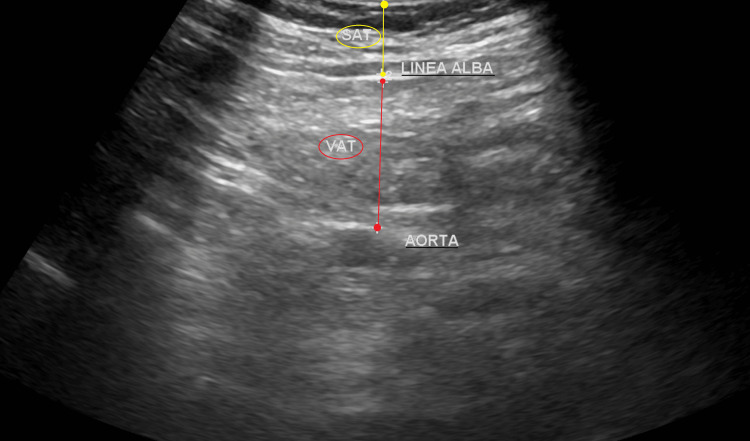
Ultrasound image shows the measurement of the VAT and SAT in a 10-year-old female child. VAT, visceral adipose tissue, SAT, subcutaneous adipose tissue
